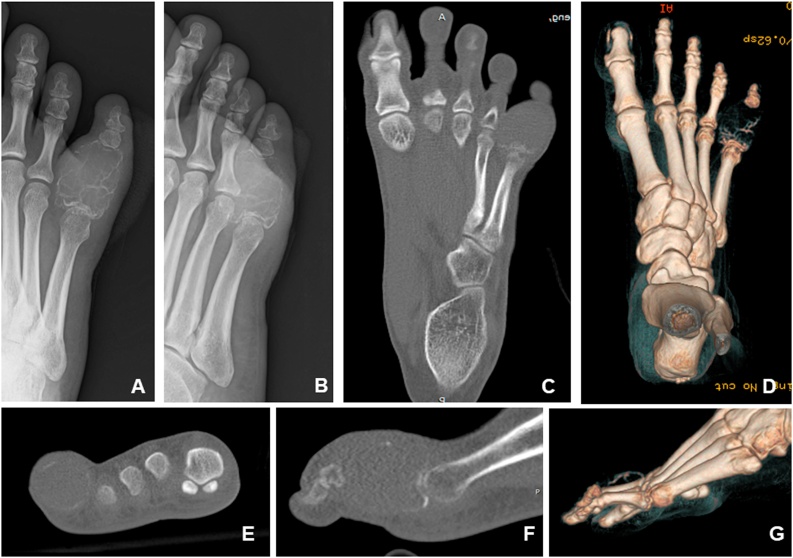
Preoperative imaging. Radiographs (Fig. 1A and B) show an expansile, osteolytic lesion of the proximal phalanx of the right fifth toe. Computed tomography (CT and 3D CT; Fig. 1C–G) show destruction of the cortex with soft tissue expansion and intra-articular invasion.
